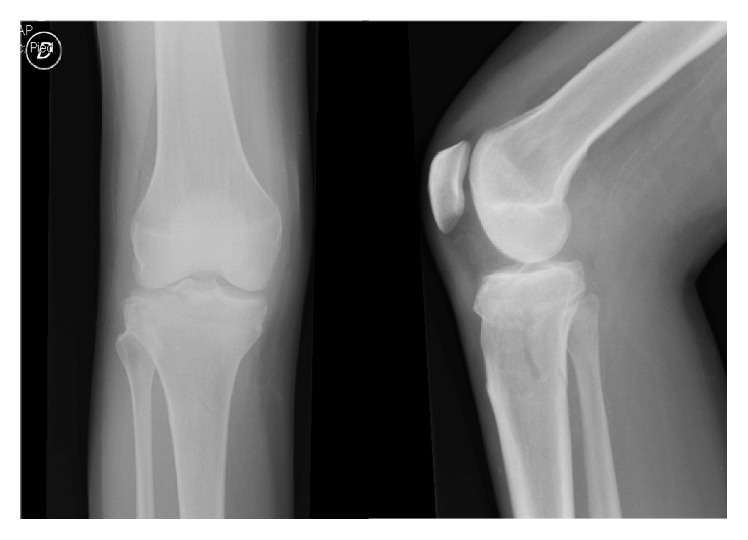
Initial X-rays of the right knee.
